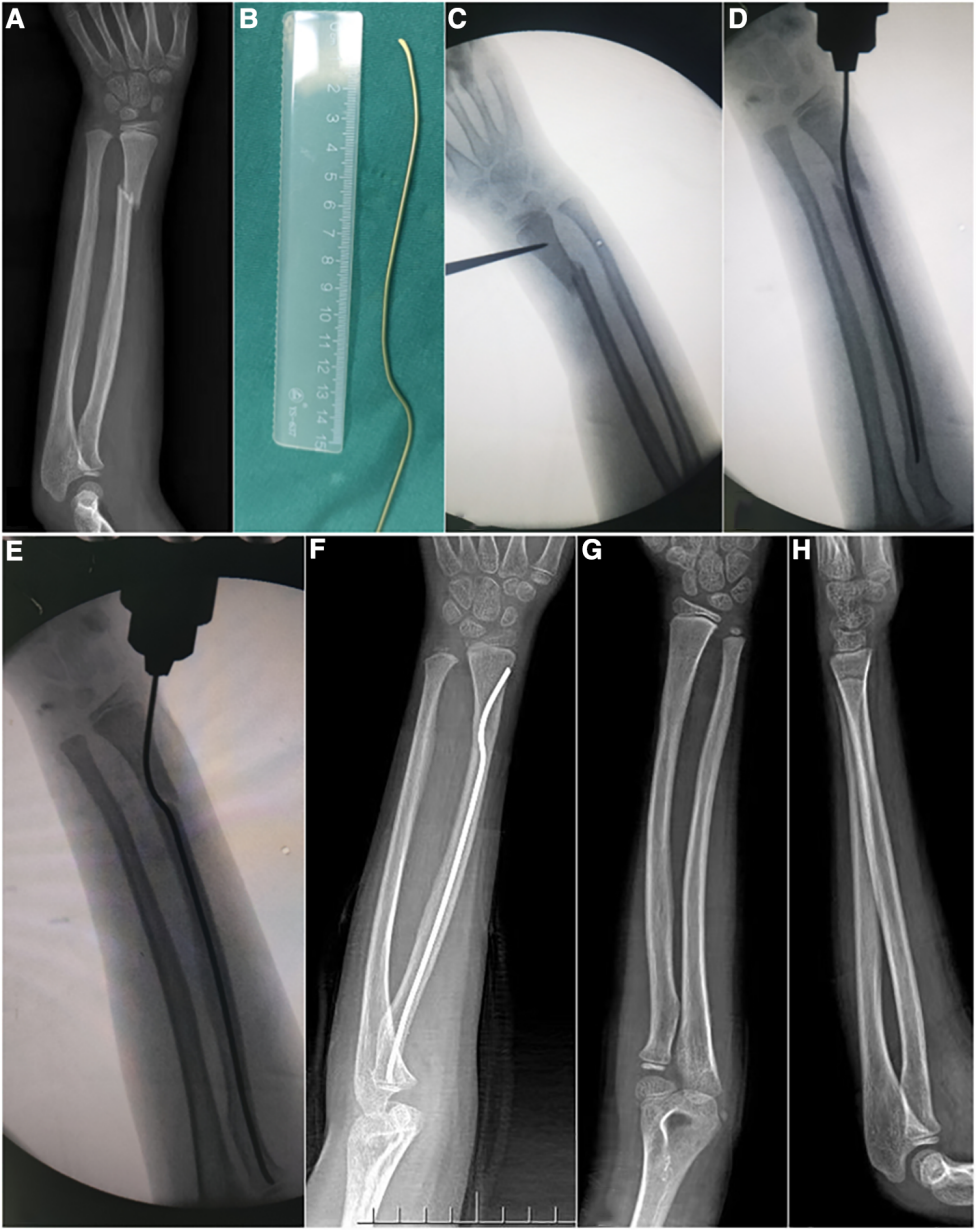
A 7-year-old child with DRMDJ fracture of the left radius. (A) x-ray film shows DRMDJ fracture of left radius; (B) according to the preoperative x-ray measurement, a precisely shaped ESIN should be pre-bended; (C) intraoperative positioning of the insertion point; (D) the displacement of the broken end is aggravated by the insertion of the unbending ESIN; (E) precise shaping ESIN is placed, and radial displacement disappears; (F) three months after the operation, the fracture had healed well; (G,H) after removing the elastic intramedullary nail 6 months after the operation, the radius was completely healed.
